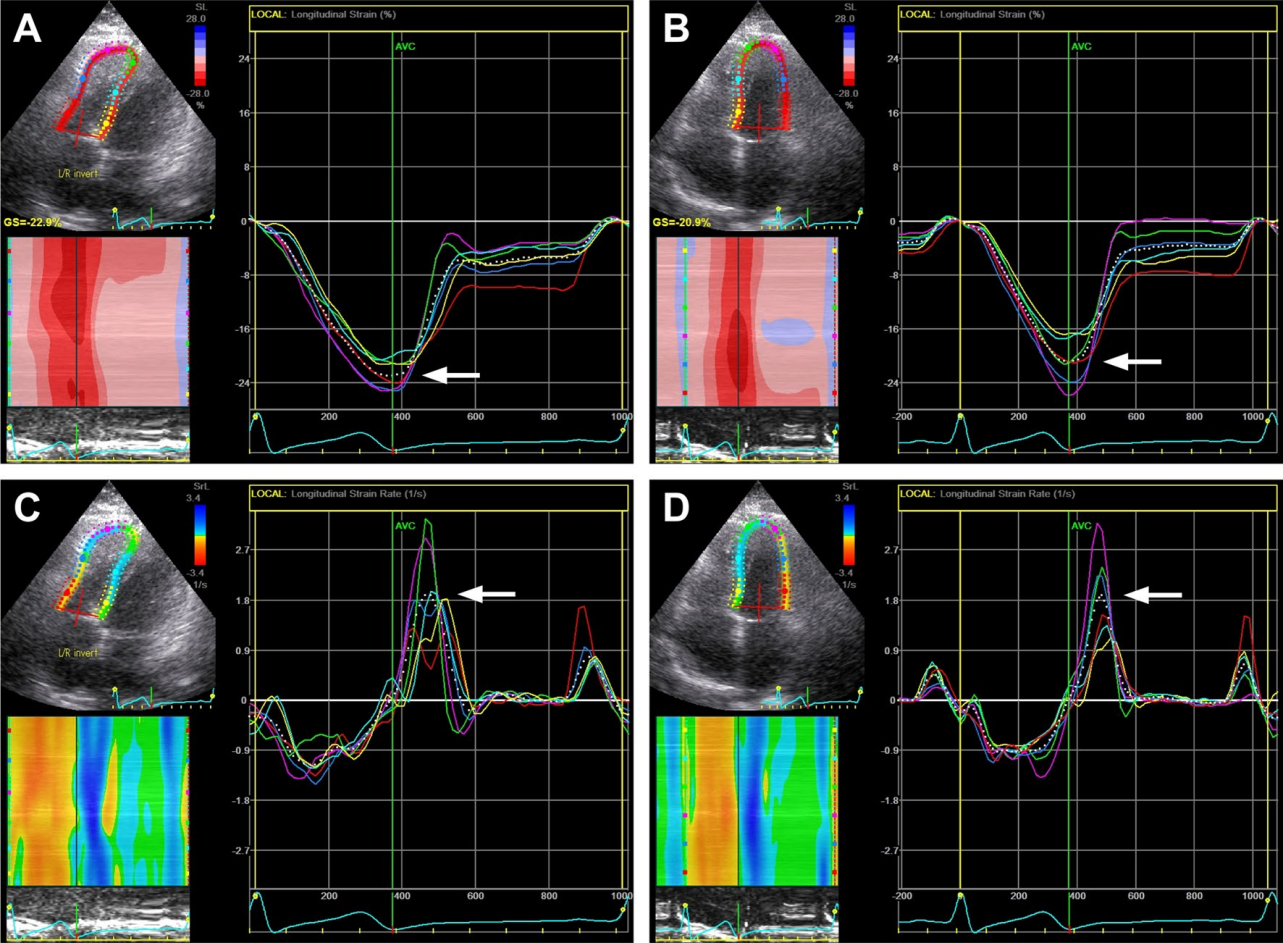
Two-dimensional speckle-tracking echocardiography in the apical 4-chamber view. White dotted lines indicate GLS or global longitudinal strain rate (average of all segments). White arrow indicates RV GLS (a), LV GLS (b), RV SRe (c), LV SRe (d). *GLS* global longitudinal strain, *LV* left ventricular, *RV* right ventricular, *SRe* early diastolic strain rate
